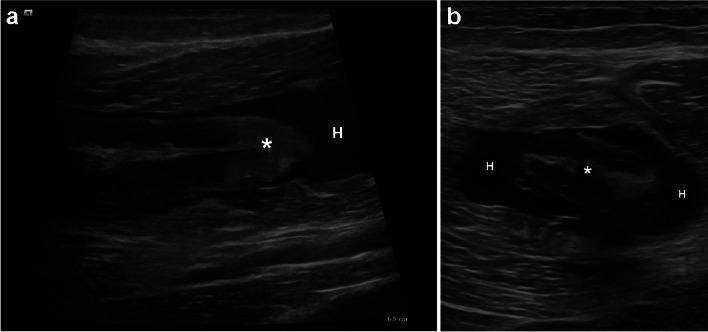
High-frequency ultrasound scan with longitudinal (A) and transverse (B) images depicting the degloving tear, with the inner muscle separated from the peripheral muscle fibers by interposed hematoma (H)
